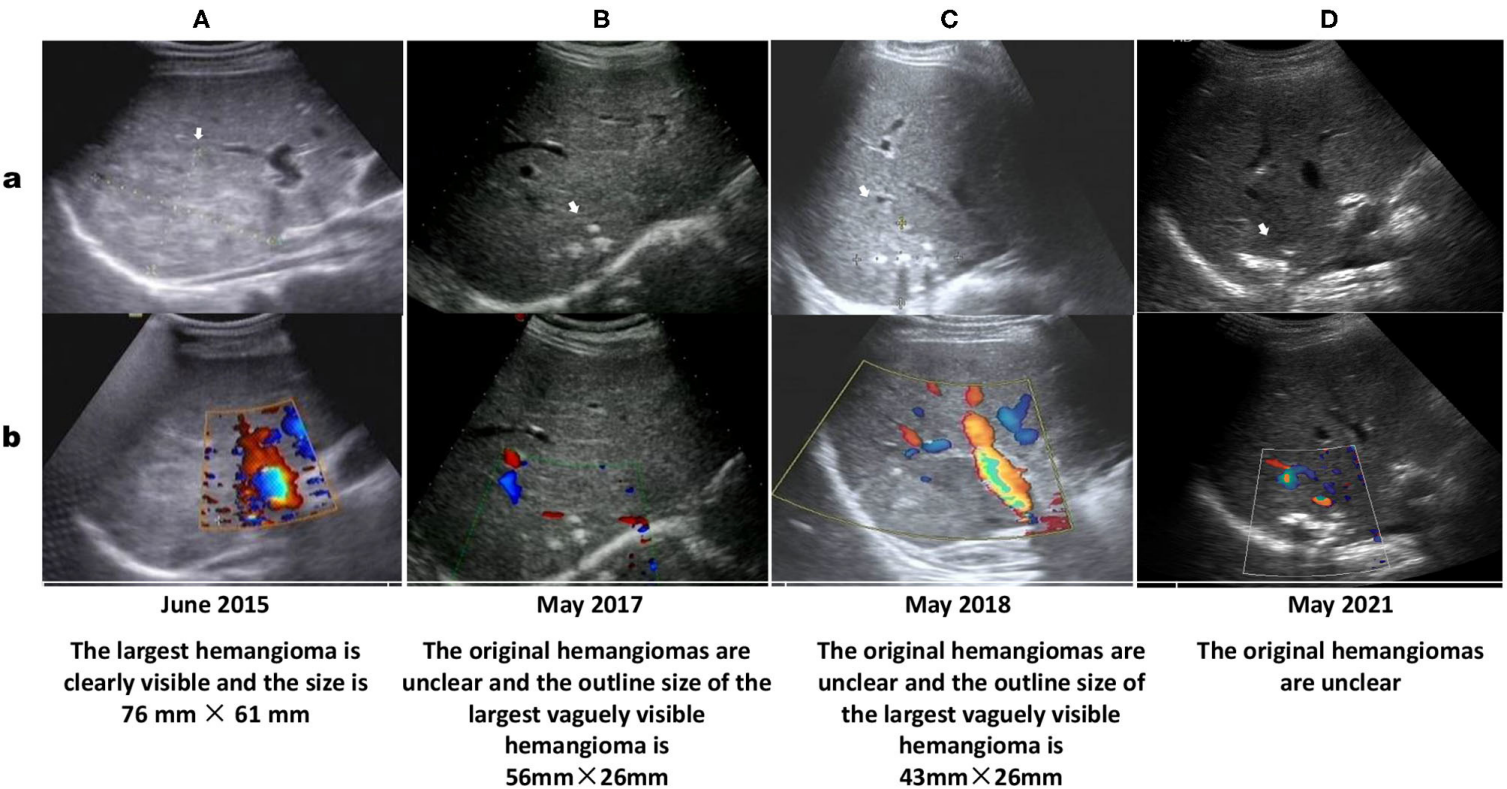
Ultrasound showing multiple hemangiomas (white arrows) that appear as echogenic nodular lesions on grayscale ultrasound (a) and non-vascular lesions on color Doppler ultrasound (b). The size of the largest segment located in the S7 segment on US performed in June 2015 (A: 76 × 61 mm) is nearly as large as that on US performed in March 2013 (according to the US report without images, 75 × 62 mm). Ultrasound images in May 2017 (B), May 2018 (C) and May 2021 (D) show that most of the multiple hepatic hemangiomas are not clearly displayed, the largest hemangioma in the S7 segment is still visible (The size described in ultrasound report performed in May 2018 is 43 × 26 mm), and calcified plaques can be seen inside.
